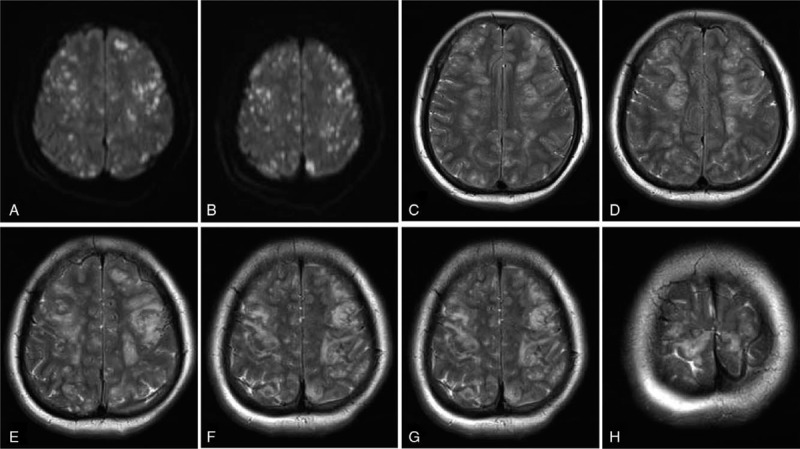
Diffusion-weighted (DW) MRI imaging (A, B) demonstrates multiple punctate foci of diffusion restriction in the gray-white junction. T2-weighted axial MRI (C–H) shows bilateral hyperintenseareas in the bilateral centrum semiovale and subcortical white matter. Images obtained 2 days after the femur fracture.
